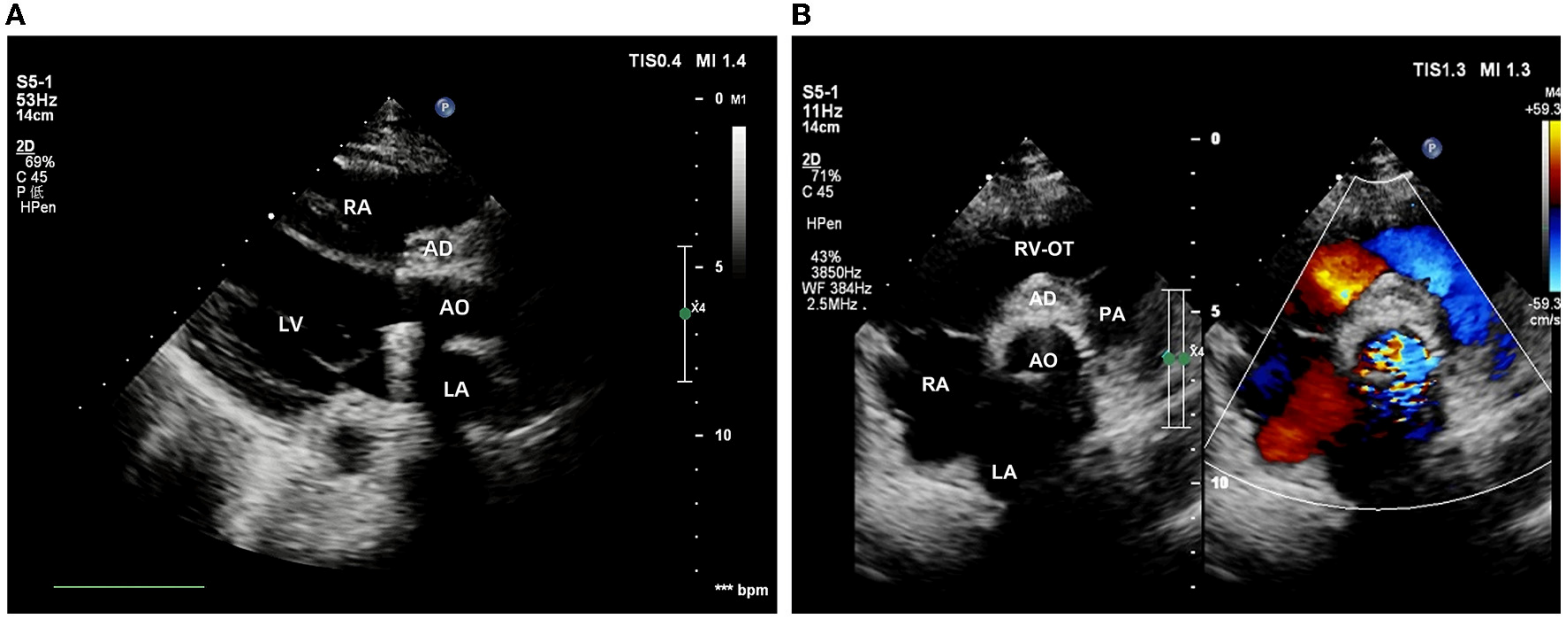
Post-operative echocardiography. (A) The position and function of the artificial valved conduit are normal. (B) The aortic root bulge is successfully closed without an internal blood flow signal.
